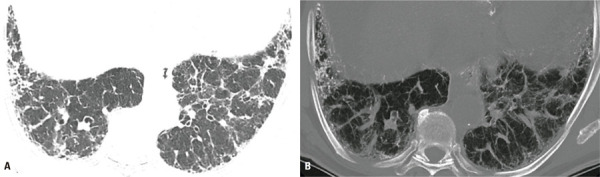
A 70-year-old man with idiopathic pulmonary fibrosis. (A) Axial chest computed tomography image showing fibrosing interstitial lung disease with a probable usual interstitial pneumonia pattern, and (B) Window-adapted maximum intensity projection showing small clustered and branching calcified nodules attributable to dendriform pulmonary ossification
